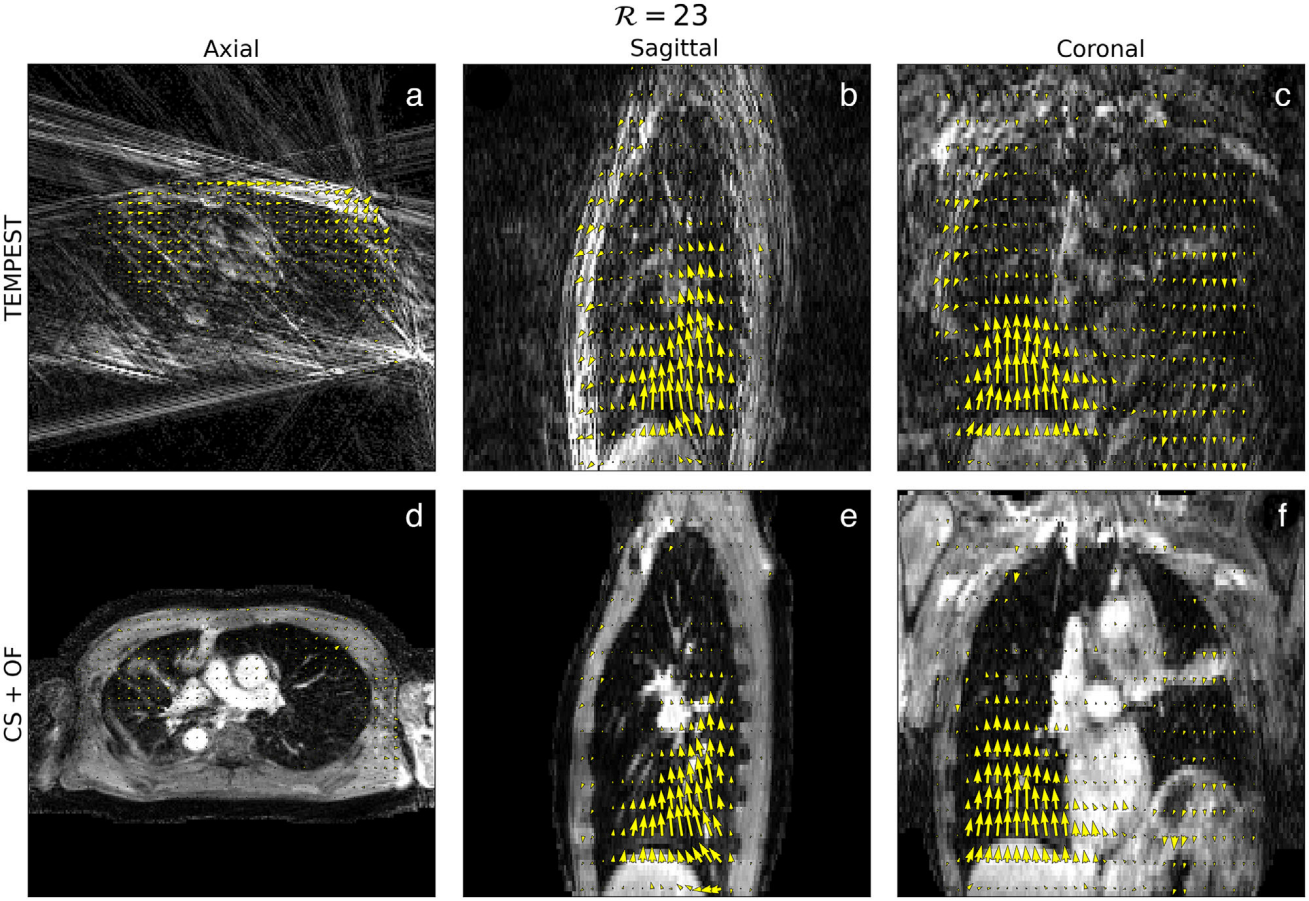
Example of motion estimation. An example of motion estimation in 3D by TEMPEST (a–c) on 23‐fold undersampled MRI. Good correspondence can be observed between the motion estimated by TEMPEST and motion estimated by optical flow computed on compressed sense reconstructions (d–f). In the quasi‐static region, TEMPEST estimates slightly larger residual motion. In the Supporting Information Videos S1–3, animated figures are provided
